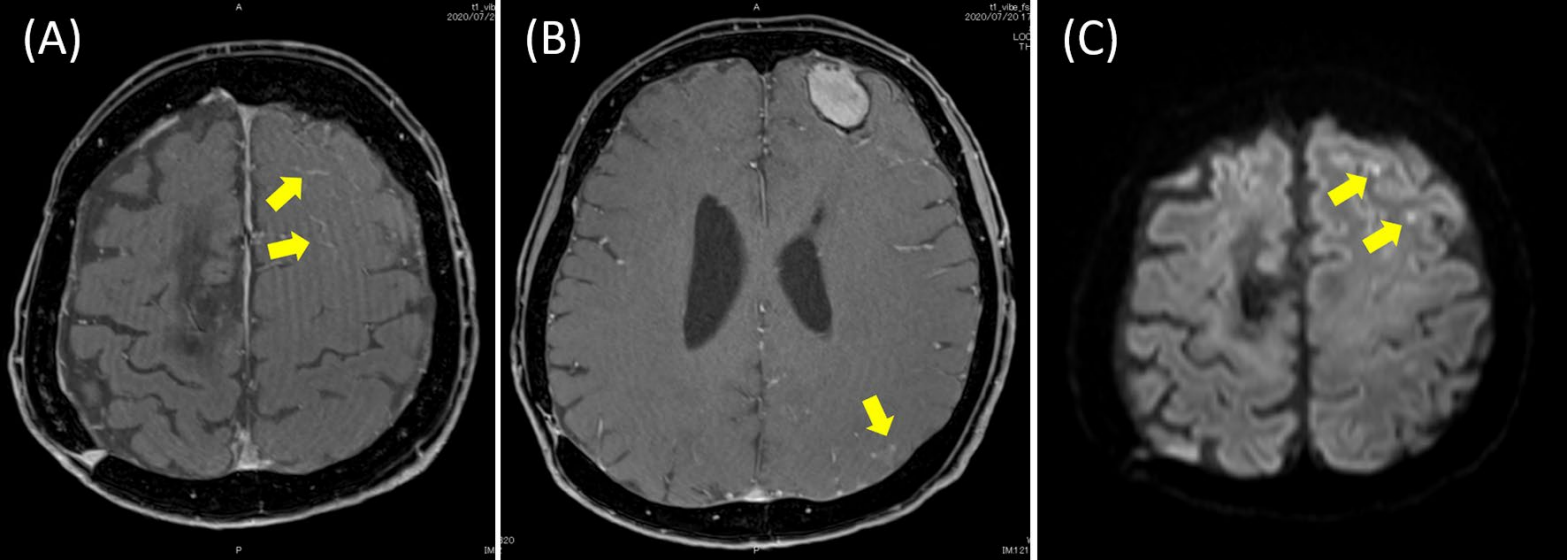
Contrast-enhanced MRI findings. A The T1-weighted image showed hyperintensity on the anterior left frontal lobe and lateral floor of the left temporal lobe (yellow arrows), with edema in the surrounding tissue. B The cerebral surface of the pia mater was slightly contrast-enhanced (yellow arrow), but there was no apparent enhancement in the cerebral parenchyma. A cystic lesion located in the anterior left frontal lobe indicates a postoperative change of meningioma as described in Fig. 1A. C A small number of minimum hemosiderin deposits (yellow arrows) were observed near the cortex, but no findings strongly suggestive of amyloid angiopathy were observed
